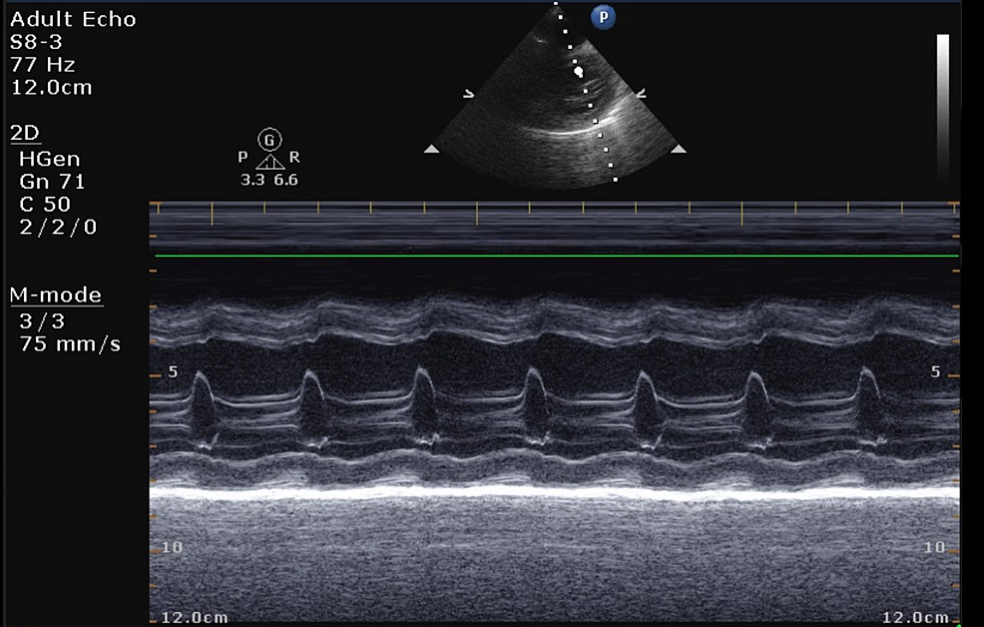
Transthoracic echocardiogram (TTE) parasternal short-axis M mode showing decreased left ventricular systolic function
